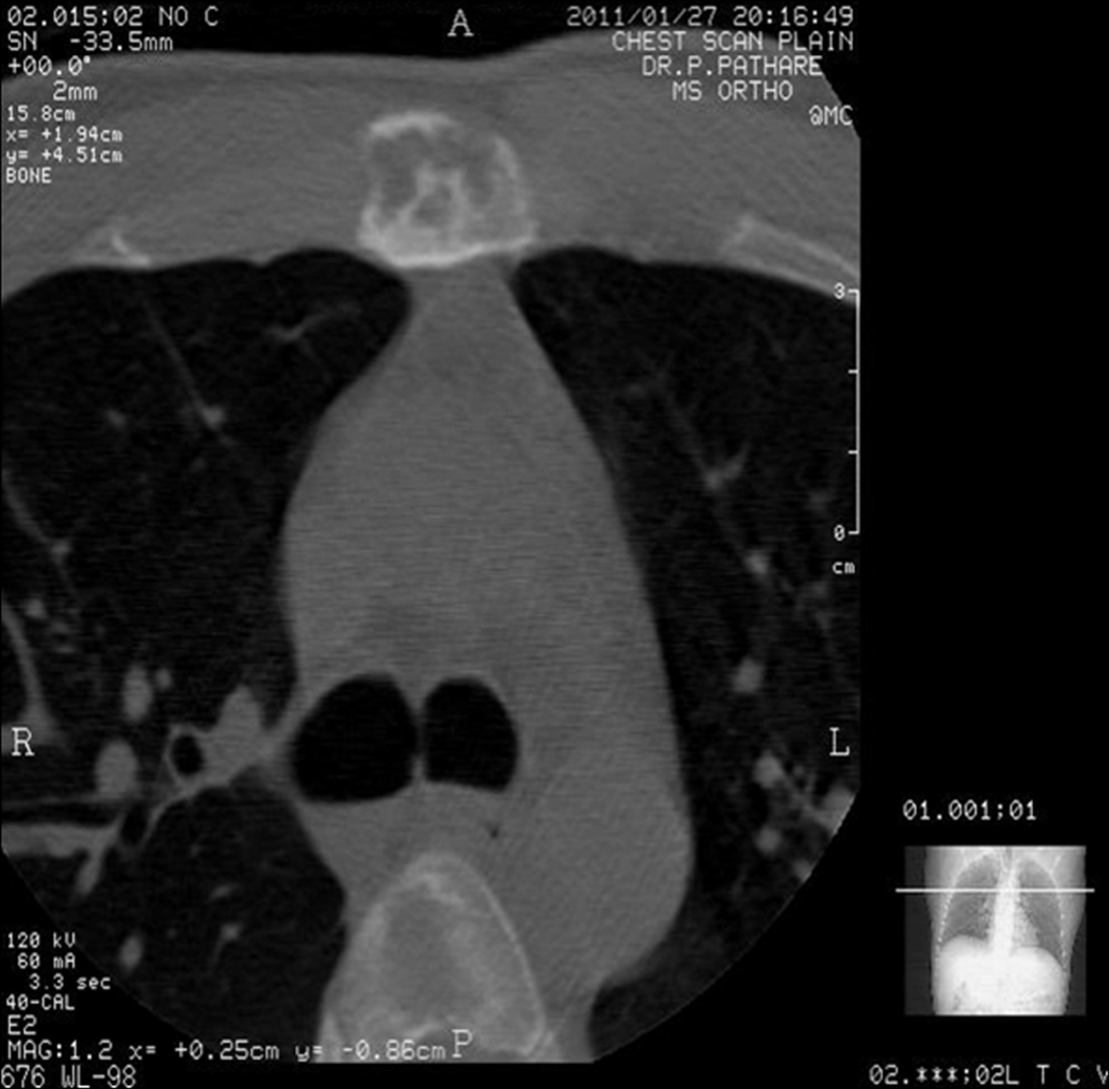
CT scan of the chest showing cavitation and multiple cyst formation in the manubriosternal joint.
